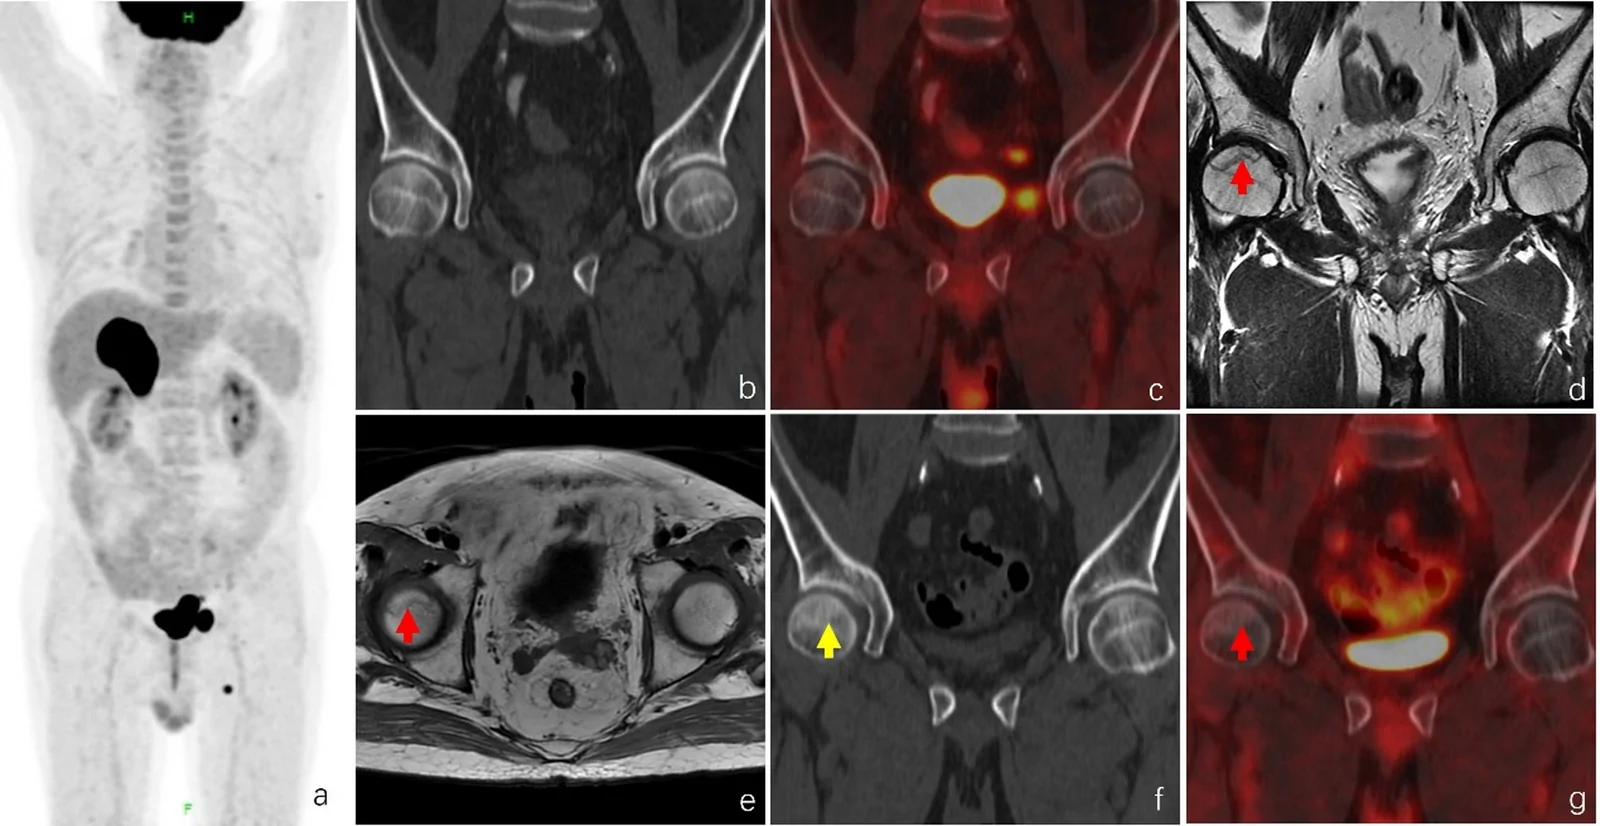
Osteonecrosis of the femoral head. PET/CT and MRI images from a 64-year-old male (No. 6) with diffuse large B-cell lymphoma after 4 cycles of chemotherapy. No abnormal findings were identified in the bilateral femoral heads on the maximum intensity projection (a), CT (b) and PET/CT fused image (c). However, an MRI scan performed on the same day as the PET/CT revealed an adipose-like high signal surrounded by linear hypointensity (arrow) at the right femoral head on the T2-weighted (d) and T1-weighted (e) images. According to the modified 2019 version of the Association Research Circulation Osseous international classification of osteonecrosis staging system, the osteonecrotic lesion of the right femoral head was classified as stage 1. A follow-up PET/CT was performed 46 months later and demonstrated a linear hyperdensity (arrows) at the right femoral head on CT (f) and PET/CT fused image (g)
